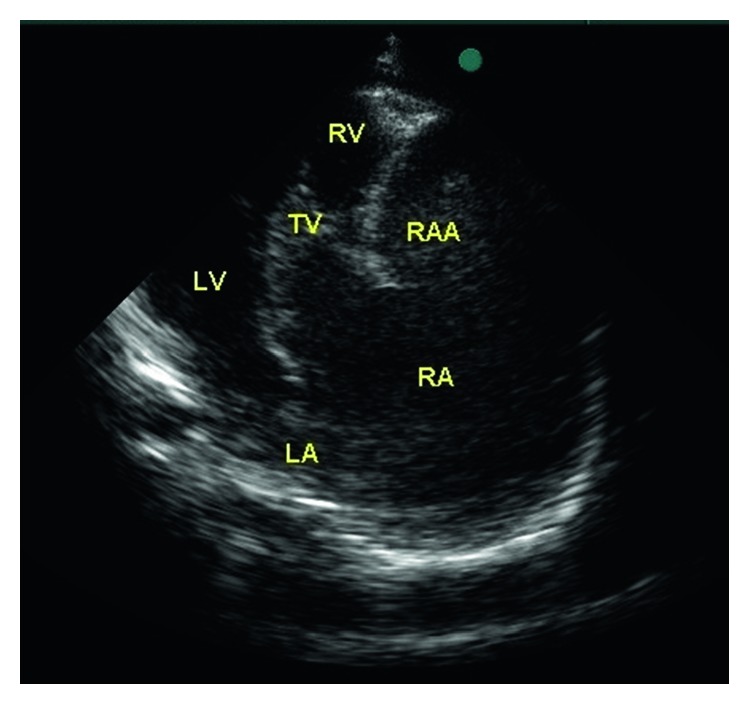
Transthoracic echocardiography of giant right atrial aneurysm associated with the right atrium. LA: left atrium, LV: left ventricle, RA: right atrium, RAA: right atrial aneurysm, RV: right ventricle, TV: tricuspid valve.
